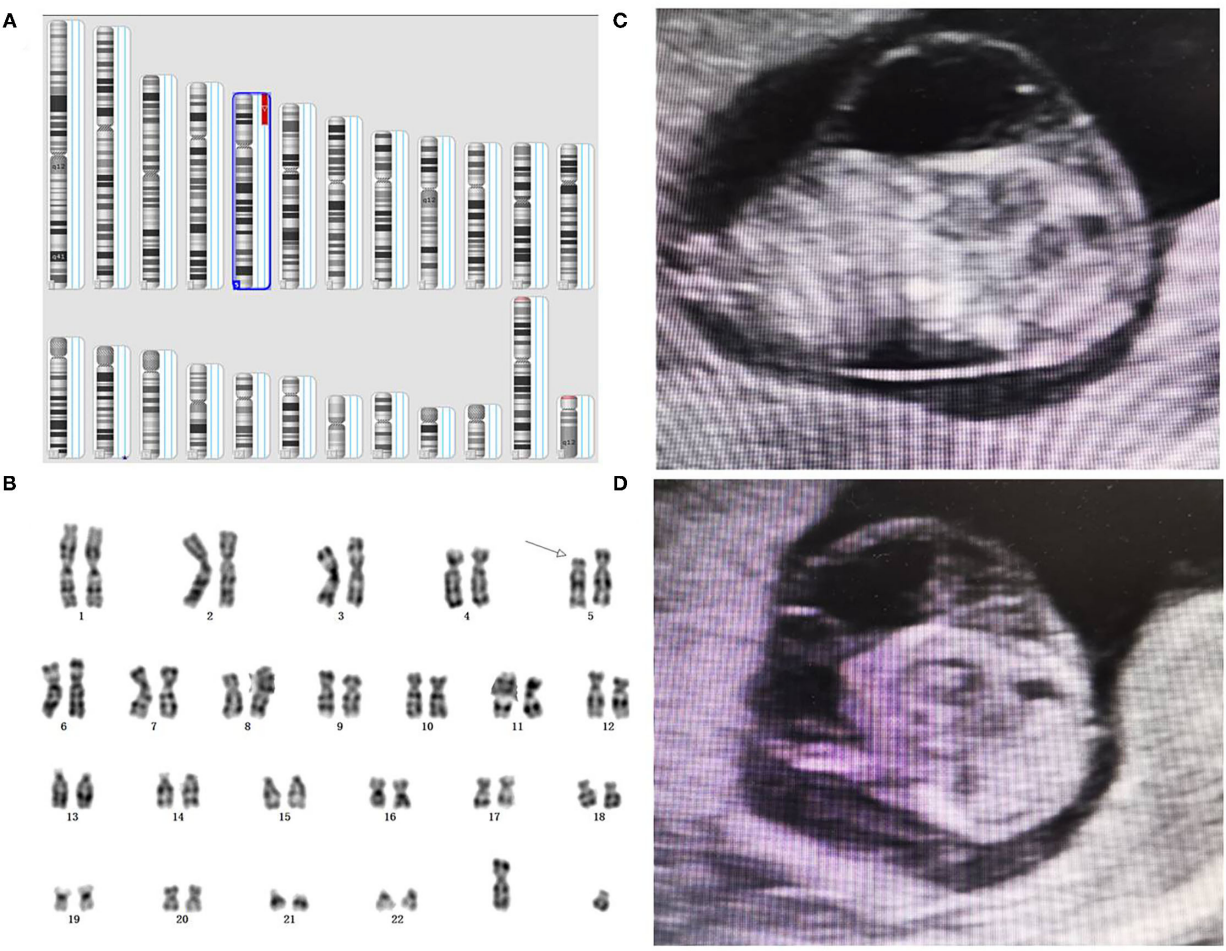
Genetic diagnosis and ultrasound phenotype of partial 5p deletion. (A) A detailed view from an SNP array analysis report for chromosomes involved. (B) Karyotype analysis revealed a partial 5p deletion in the fetus. (C, D) Prenatal ultrasound elicited cystic hygroma colli in the fetus.
